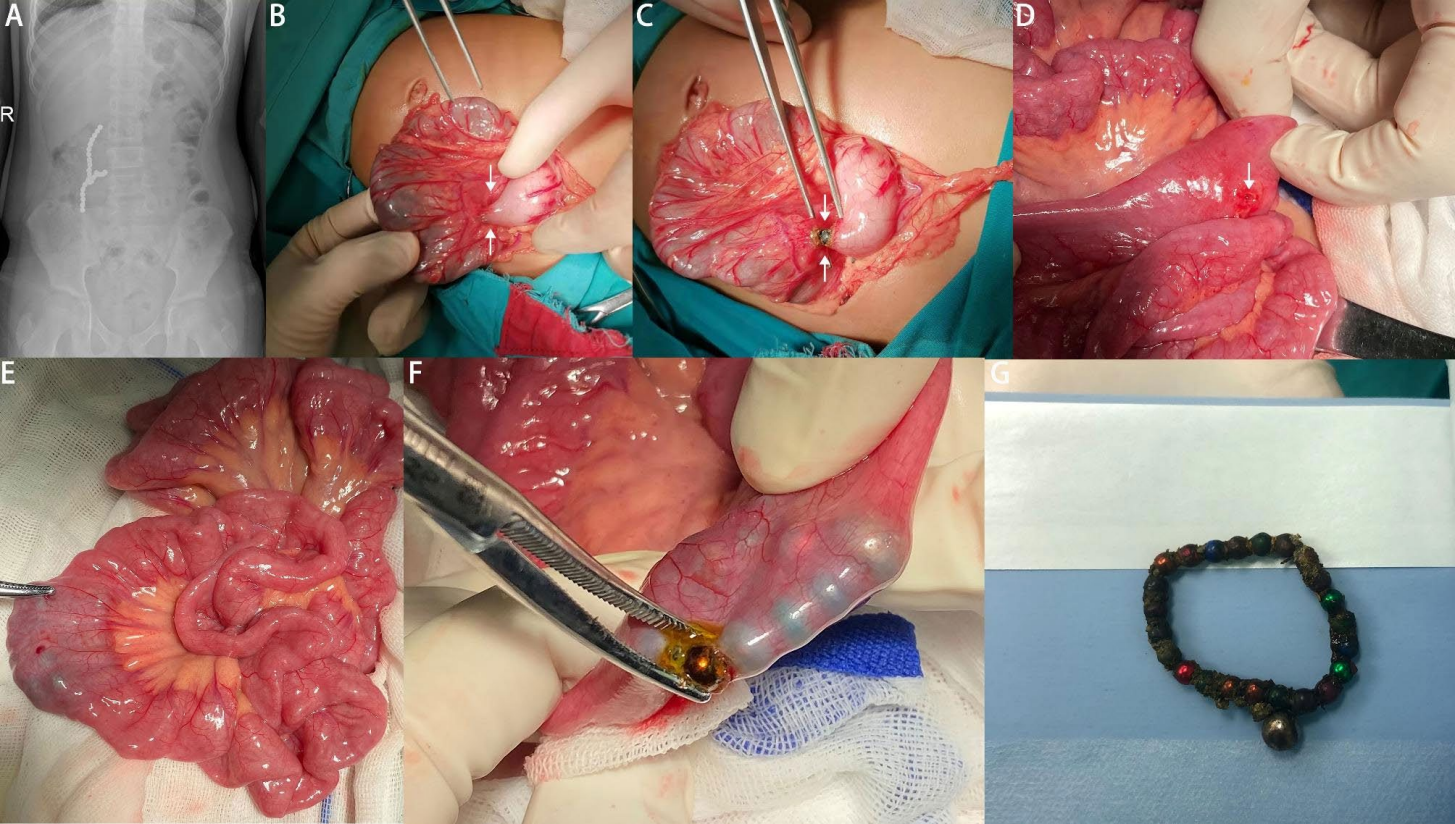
Diagnostic and surgical procedure. The surgical methods that were used for the removal of foreign bodies in the gastrointestinal tract. (A: abdominal upright X-ray; B: The two arrows point to an internal fistula; C: The two arrows point to a magnetic bead after incision of the fistula; D: The arrow points to a perforation of the intestine; E: The forceps is attracted by magnetic beads in the intestinal cavity; F: Magnetic beads after incision of intestinal wall; G: Magnetic beads after removal)
